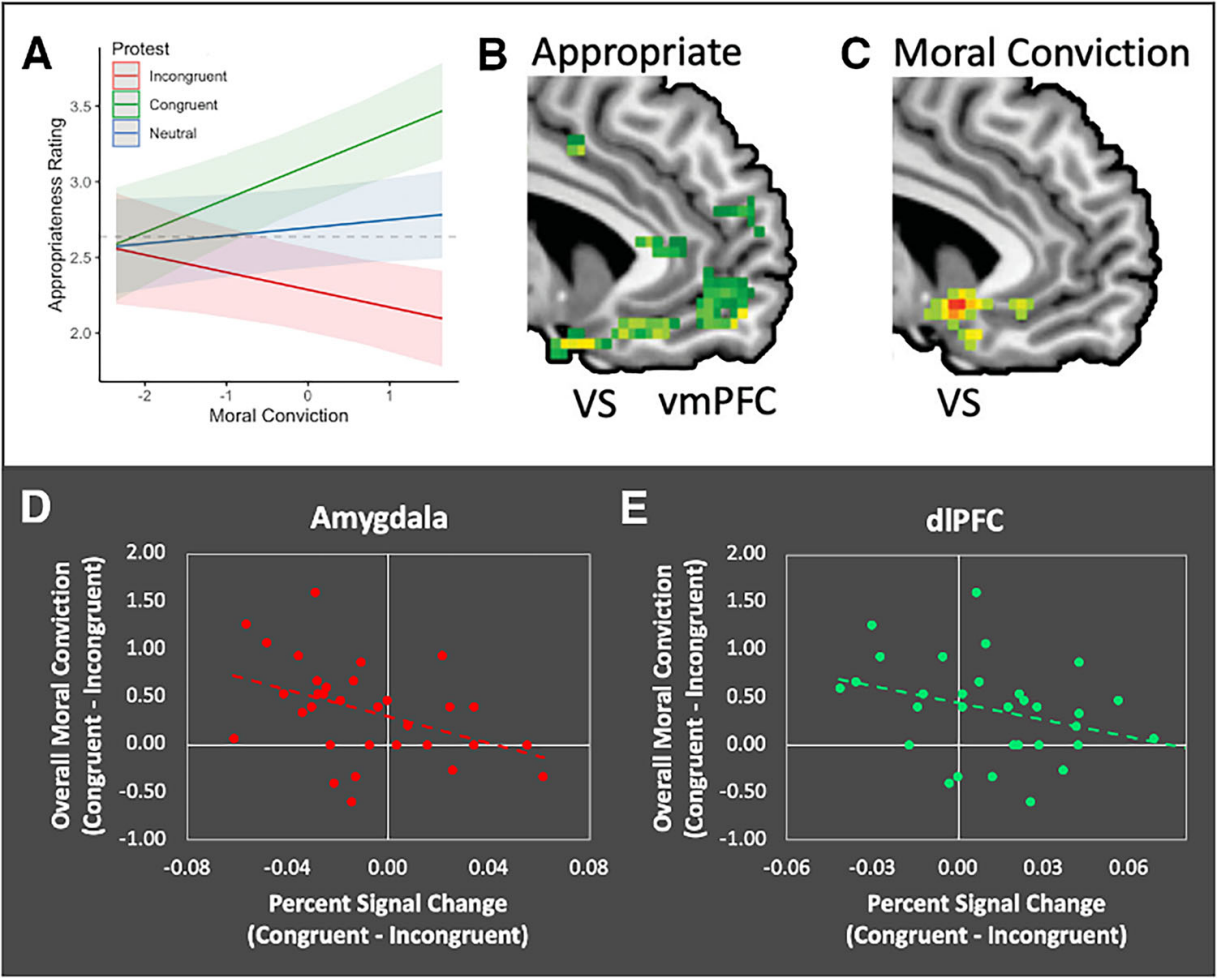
Neural response to the congruency of violent political protests. In this study, college students reported their moral convictions about a variety of sociopolitical issues (e.g., Black Lives Matter, abortion rights, ecological transition) prior to undergoing functional MRI scanning. During scanning, they were asked to evaluate the appropriateness of violent protests that were ostensibly congruent or incongruent with their views about sociopolitical issues. (A) An interaction between congruency and moral conviction predicted appropriateness ratings. Parametric increases in neurohemodynamic signal in the ventromedial prefrontal cortex (vmPFC) and ventral striatum (VS) predicted (B) appropriateness ratings and (C) the intensity of participants’ moral convictions, for congruent causes. When participants showed greater overall moral conviction (the difference in mean moral conviction for congruent vs. incongruent issues), decrease in response was detected for congruent issues in (D) amygdala and (E) dorsolateral prefrontal cortex (dlPFC). Adapted from Ref. [113].
